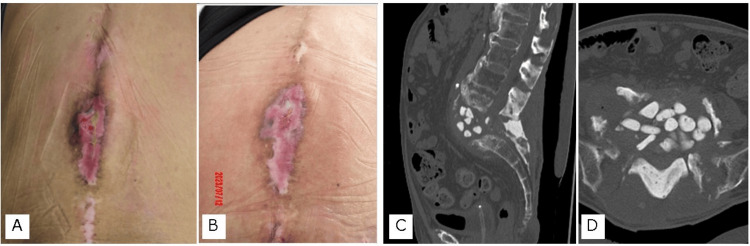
Preoperative bed sore condition and computed tomography (CT) A. Pressure ulcer at the apex of the kyphosis before the first surgery; B. Pressure ulcer at the apex of the kyphosis before the second surgery; C Lumbosacral mid-sagittal reconstruction CT before the second surgery; D. Lumbosacral axial CT at the L5/S1 level before the second surgery
